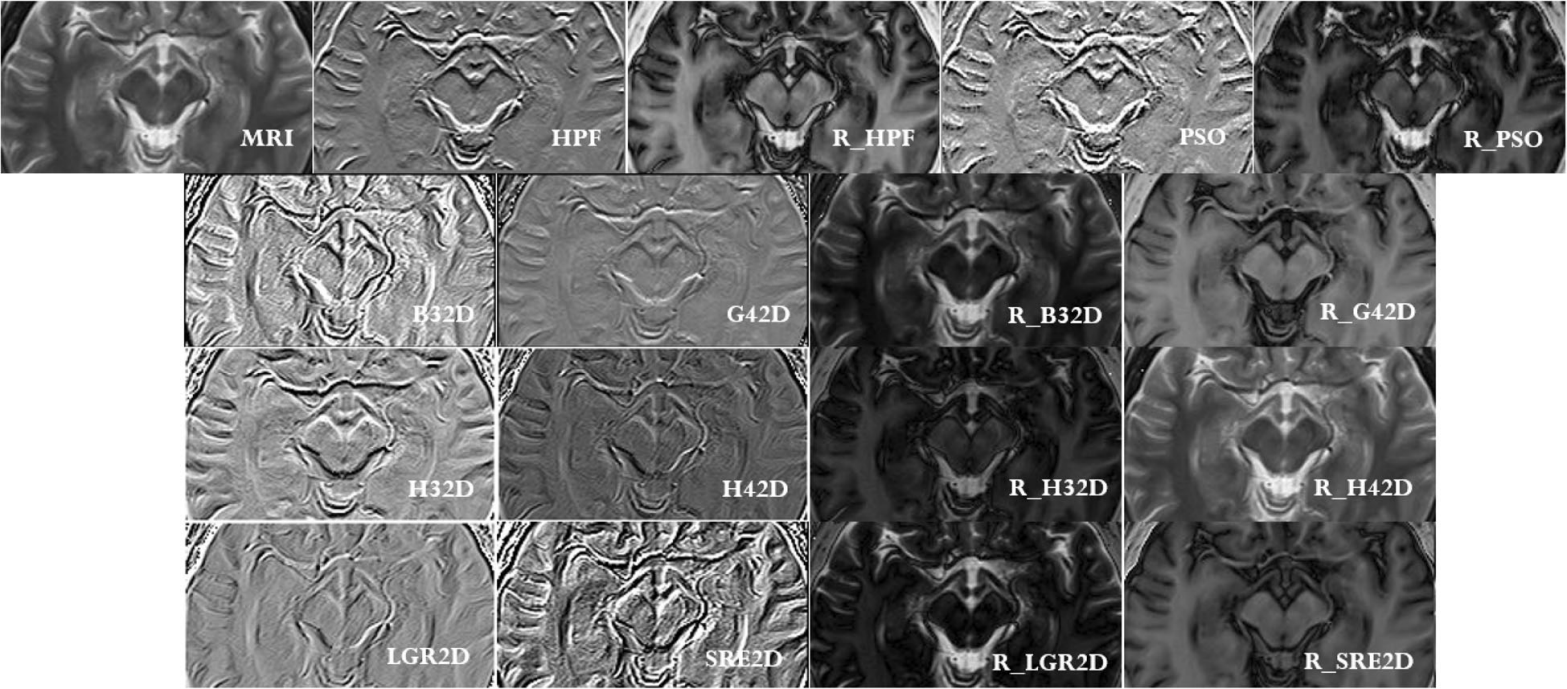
Comparative study across filtering techniques. Top row (from left to right): MRI, HPF, reconstructed MRI through HPF (R_HPF), PSO-based HPF, and reconstructed MRI through PSO (R_PSO). Second row: ICF of B32D, ICF of G42D, reconstructed MRI through ICF of B32D (R_B32D), and reconstructed MRI through ICF of G42D (R_G42D). Third row from the top: ICF of H32D, ICF of H42D, reconstructed MRI through ICF of H32D (R_H32D), and reconstructed MRI through ICF of H42D (R_H42D). Bottom row: ICF of LGR2D, ICF of SRE2D, reconstructed MRI through ICF of LGR2D (R_LGR2D), and reconstructed MRI through ICF of SRE2D (R_SRE2D)
